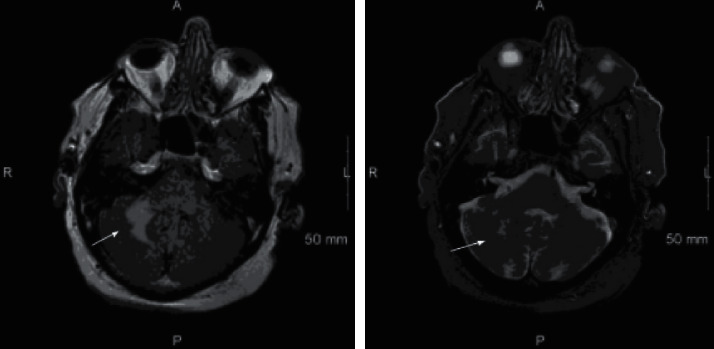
T2 and FLAIR magnetic resonance imaging (MRI) showing a hyperintense lesion without mass effect in the right cerebellar hemisphere.
